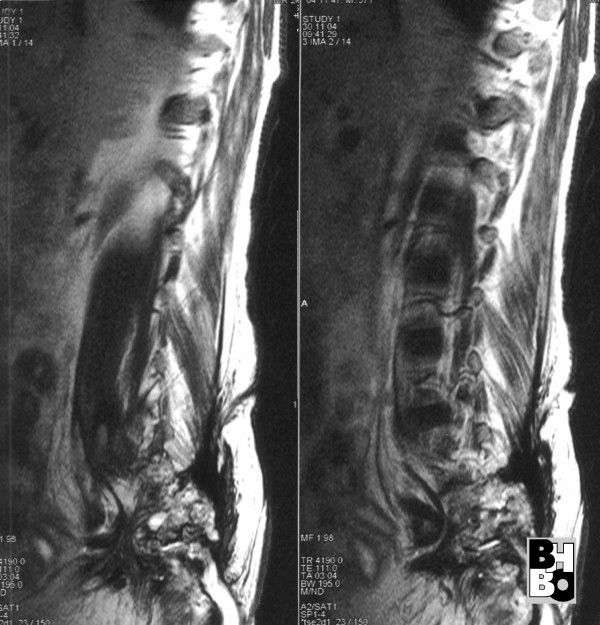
Sagital sections (MRI) of the lumbal and sacral spine showing extended osseous destructions of the sacrum.
